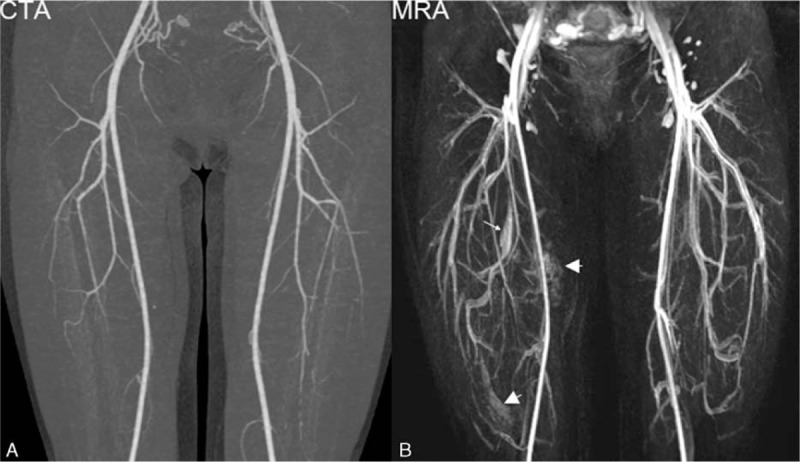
Intramuscular hemangioma. (A) An arterial abnormality is difficult to identify on the CTA MIP image. (B) Subtle perivascular enhancement (thick white arrows) and a dilated draining vein (thin white arrow) on MRA are consistent with the presence of a vascular malformation. CTA = computed tomography angiography, MRA = magnetic resonance angiography.
